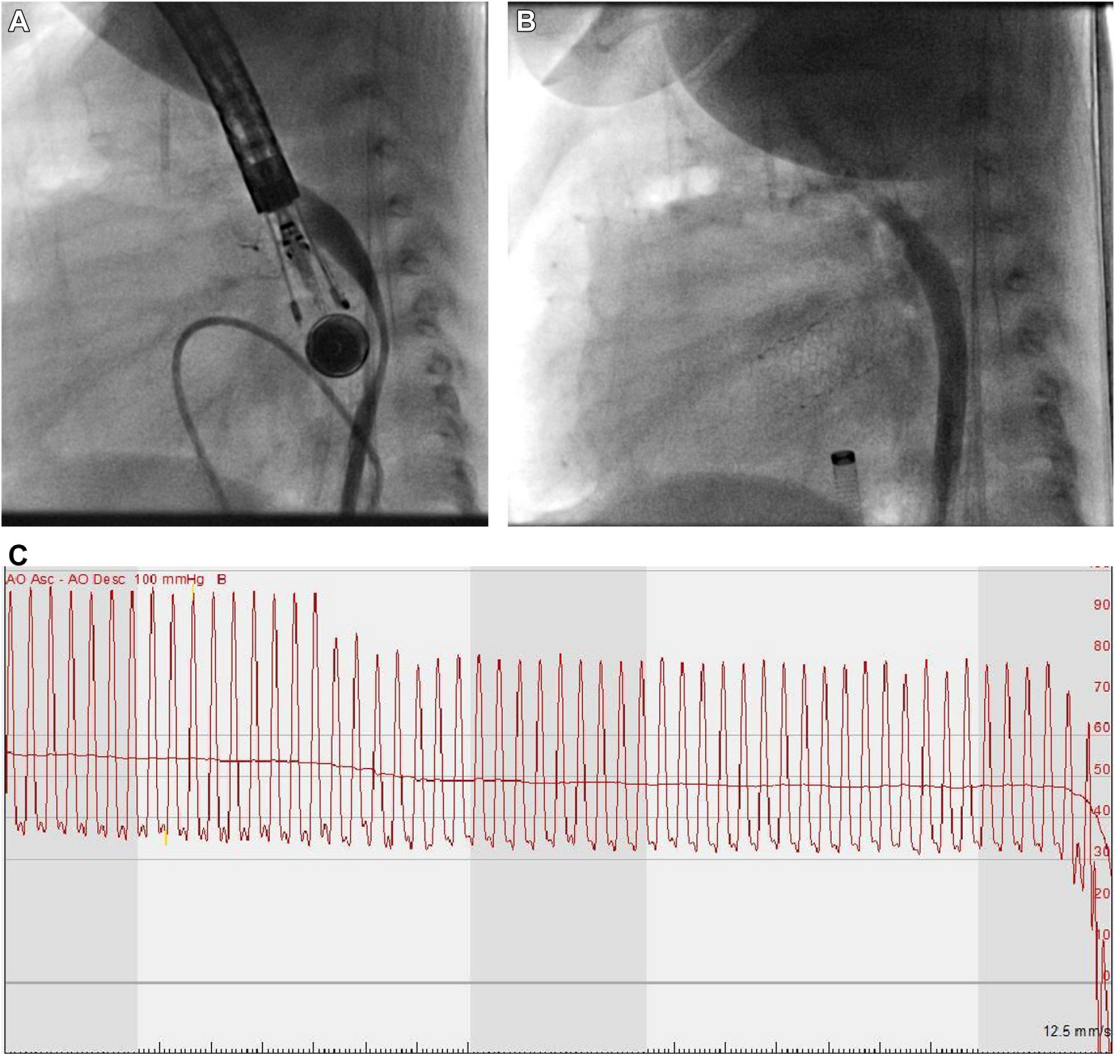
(A) Compression of the thoracic descending aorta by the TEE probe in anterior-posterior direction with near obliteration of aortic lumen. Probe dimensions: tip: 1.5 × 3.5 cm (0.6 × 1.4 in) W × L, shaft: 1.0 cm (0.4 in) diameter, 1 m (39.4 in). (B) Resolution of compression with removal of the TEE probe; contrast injection filling the widely patent thoracic descending aorta. (C) Femoral arterial waveform at the time of advancement of the TEE probe to mid-esophageal level with ensuing profound hypotension. TEE, transesophageal echocardiography.
